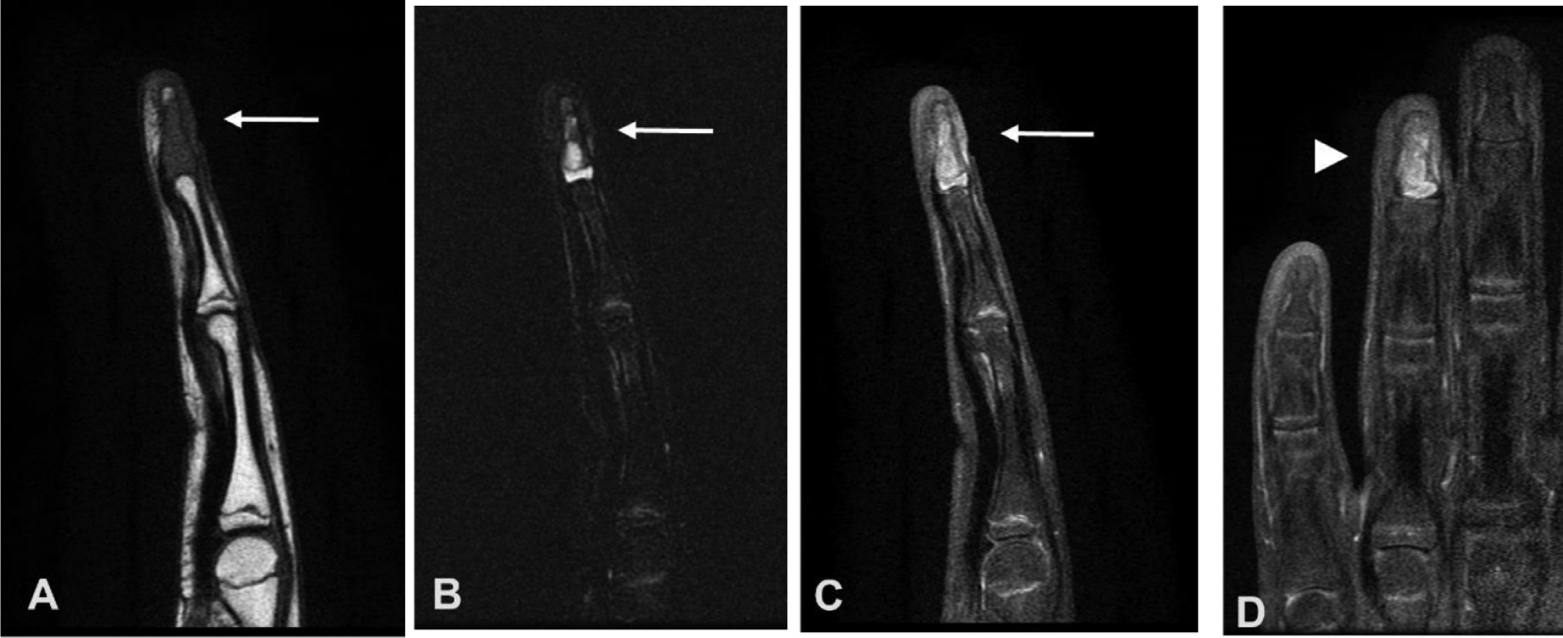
(A) Sagittal T1-weighted nonfat-suppressed MRI of the left hand demonstrates a hypointense lesion (arrow) involving the fourth digit distal phalanx, with slight sparing of the tuft and no cortical disruption. (B) Sagittal T2-weighted fat saturated and (C) T1-weighted fat saturated post contrast MRI of the left hand demonstrates heterogeneous increased signal intensity involving the bone (arrow) without abnormal soft tissue signal. (D) Coronal T1-weighted fat-saturated postcontrast images of the third through fifth digits demonstrates mild enhancement of the lesion (arrow head).
